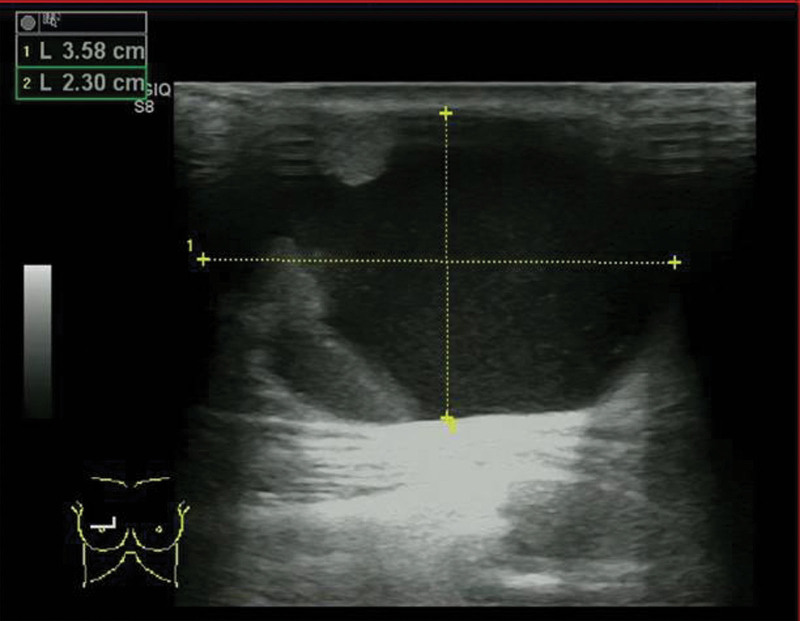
On mammary ultrasonography, a well-defined predominantly cystic-solid lesion of 3.6 × 2.3 cm was confirmed immediately below the right papilla (BI-RADS grade 3); a solid areas with a gradual rise from the cyst wall was confirmed within the cyst.
